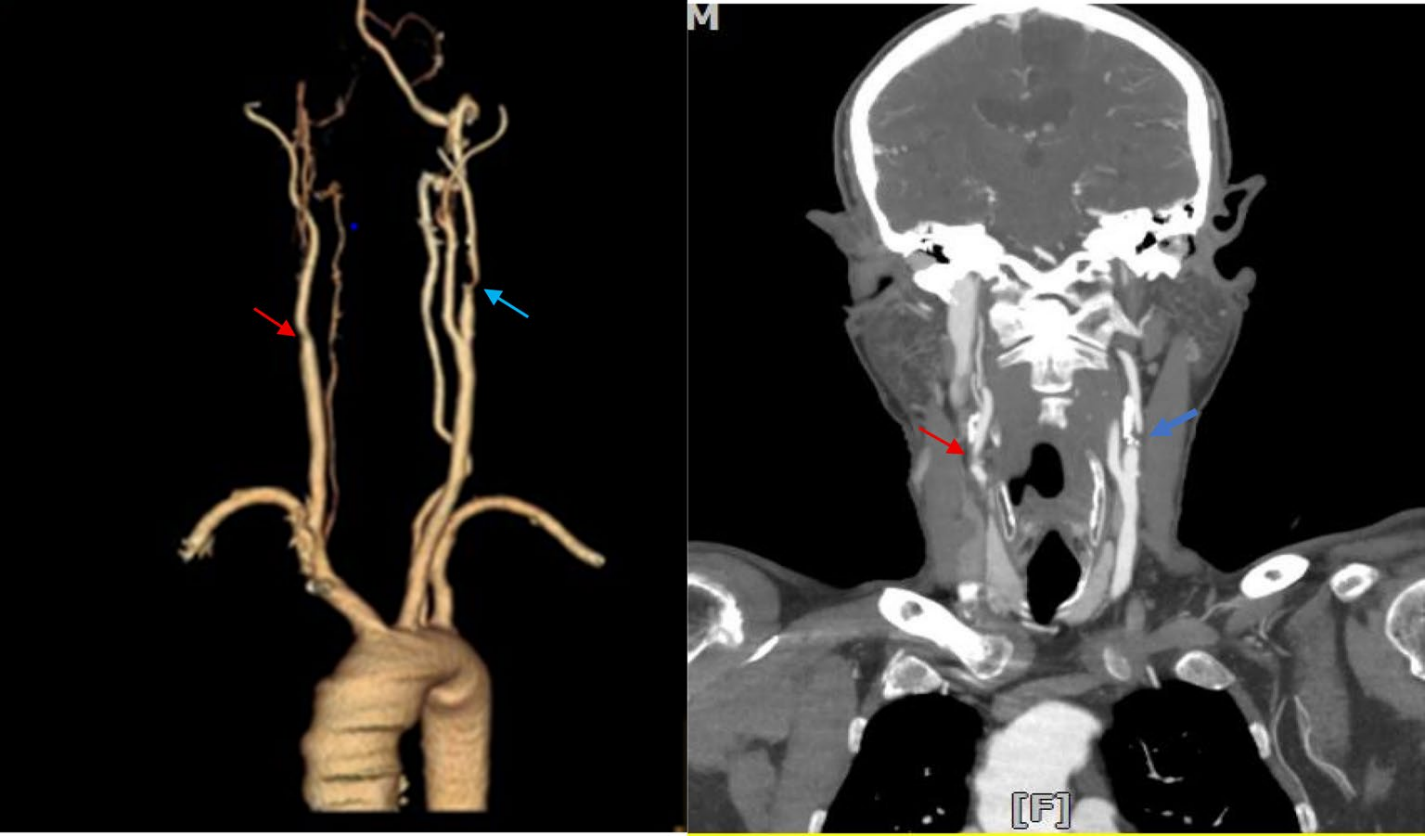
Preoperative CT angiography (CTA) image showing 99% stenosis in the right common and internal carotid arteries (indicated by red arrows) and the previously implanted stent in this area. Additionally, 75% stenosis is observed in the left carotid artery (blue arrow), which was associated with neurological symptoms (motor weakness in the right side of the body).
